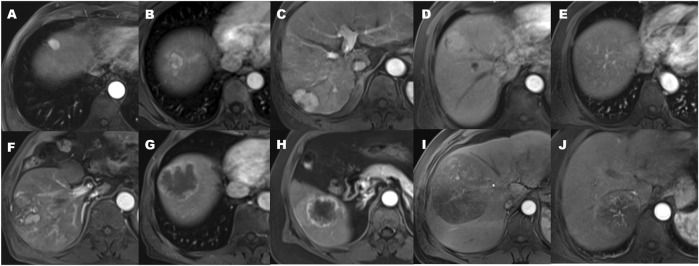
The patterns of arterial phase enhancement of hepatocellular carcinoma on contrast-enhanced MRI. (A) Homogeneously hypervascular HCC. (B) Hypervascular HCC with slit-like hypovascular foci. (C) With multifocal hypovascular foci. (D) Hypervascular HCC with the peripheral hypovascular area. (E) Completely isovascular HCC. (F) HCC with a nodular or irregular-shaped hypovascular portion at the inner area. (G) Hypovascular HCC with irregular rim-like enhancement. (H) Hypovascular HCC with the peripheral hypervascular area, discontinuous rim or crescent-like, and the presence of peritumoral enhancement seen in the arterial phase. (I) Hypovascular HCC with linear or spot-like hypervascular foci. (J) the presence of an intratumoral artery.
